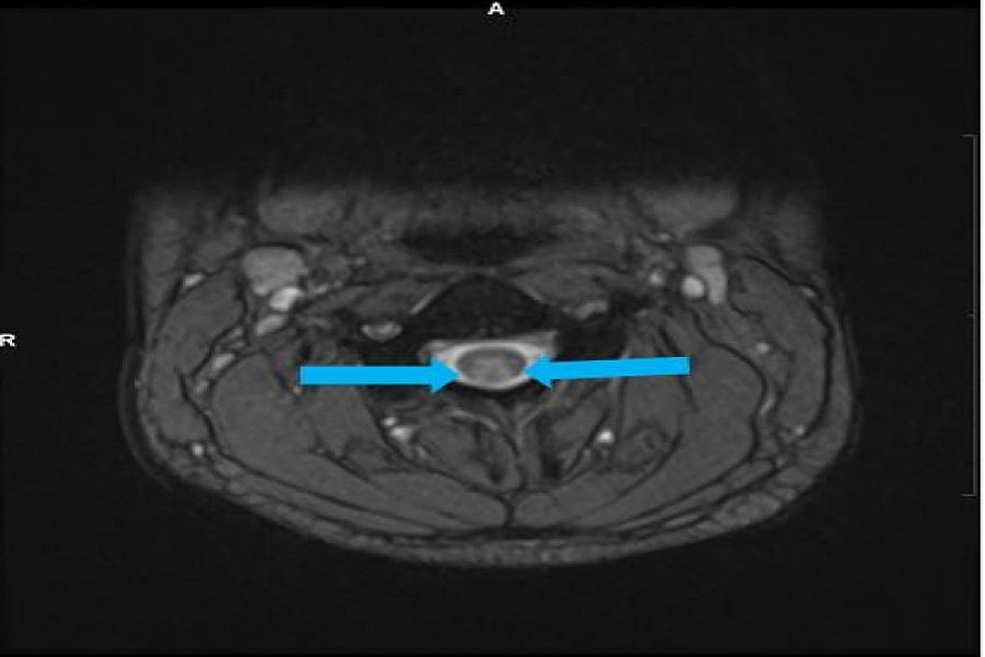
Axial MRI T2-weighted image showing abnormal bilateral high T2 cord signal in the dorsal columns over a relatively long length. The location of the signal abnormality and the length of the cord involvement are consistent with subacute combined degeneration of the cord (blue solid arrows).
